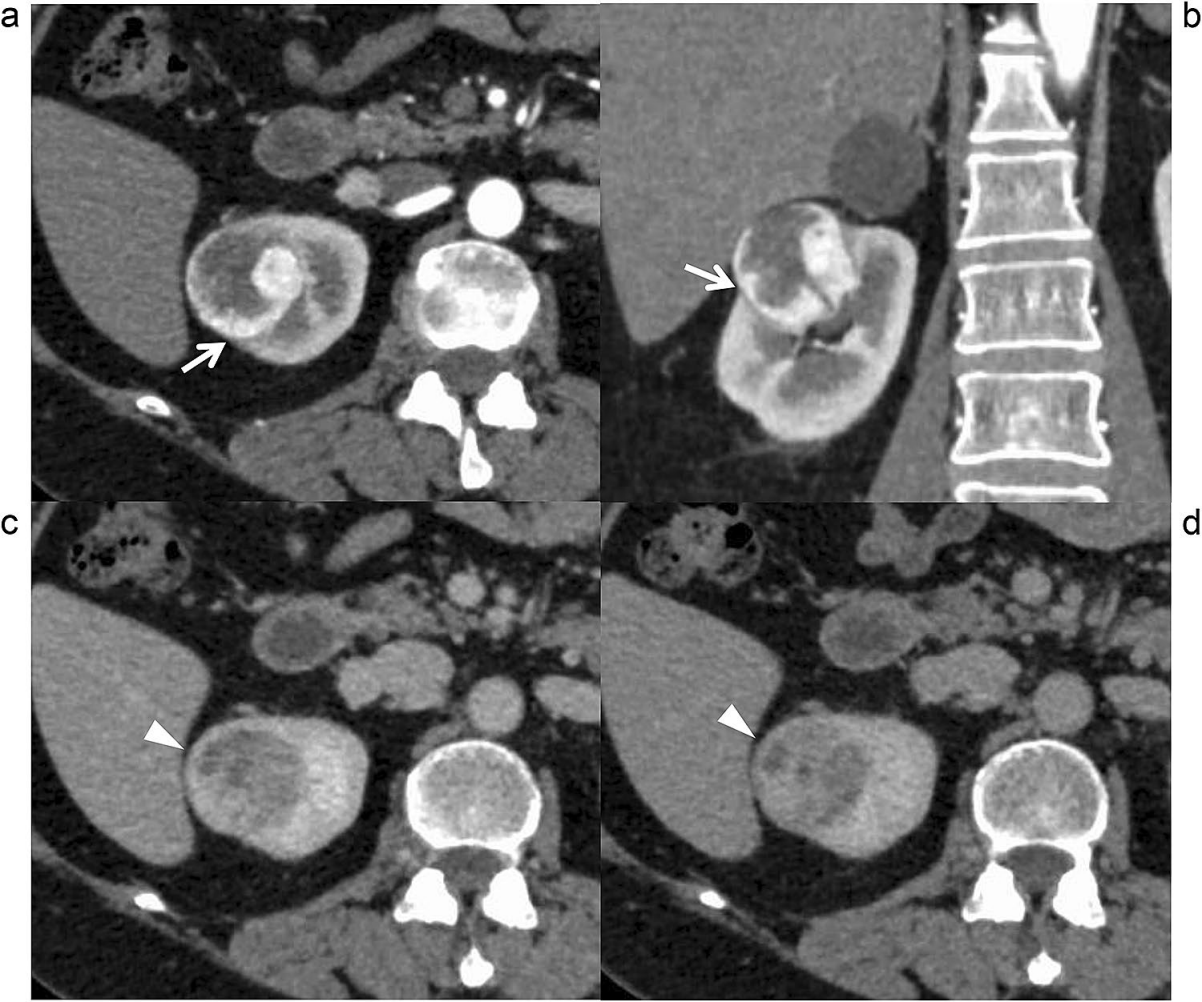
Contrast-enhanced computed tomography of a clear cell renal cell carcinoma without a pathological peritumoral pseudocapsule in a 53-year-old women. A thin low-attenuation rim is observed partly between the tumor and renal cortex (arrows) on the cortico-medullary phase trans-axial (a) and coronal images (b). A suspected high-attenuation rim is observed on the nephrographic (c) or excretory phase (d) images. These findings represent false-positive results of a pseudocapsule.
